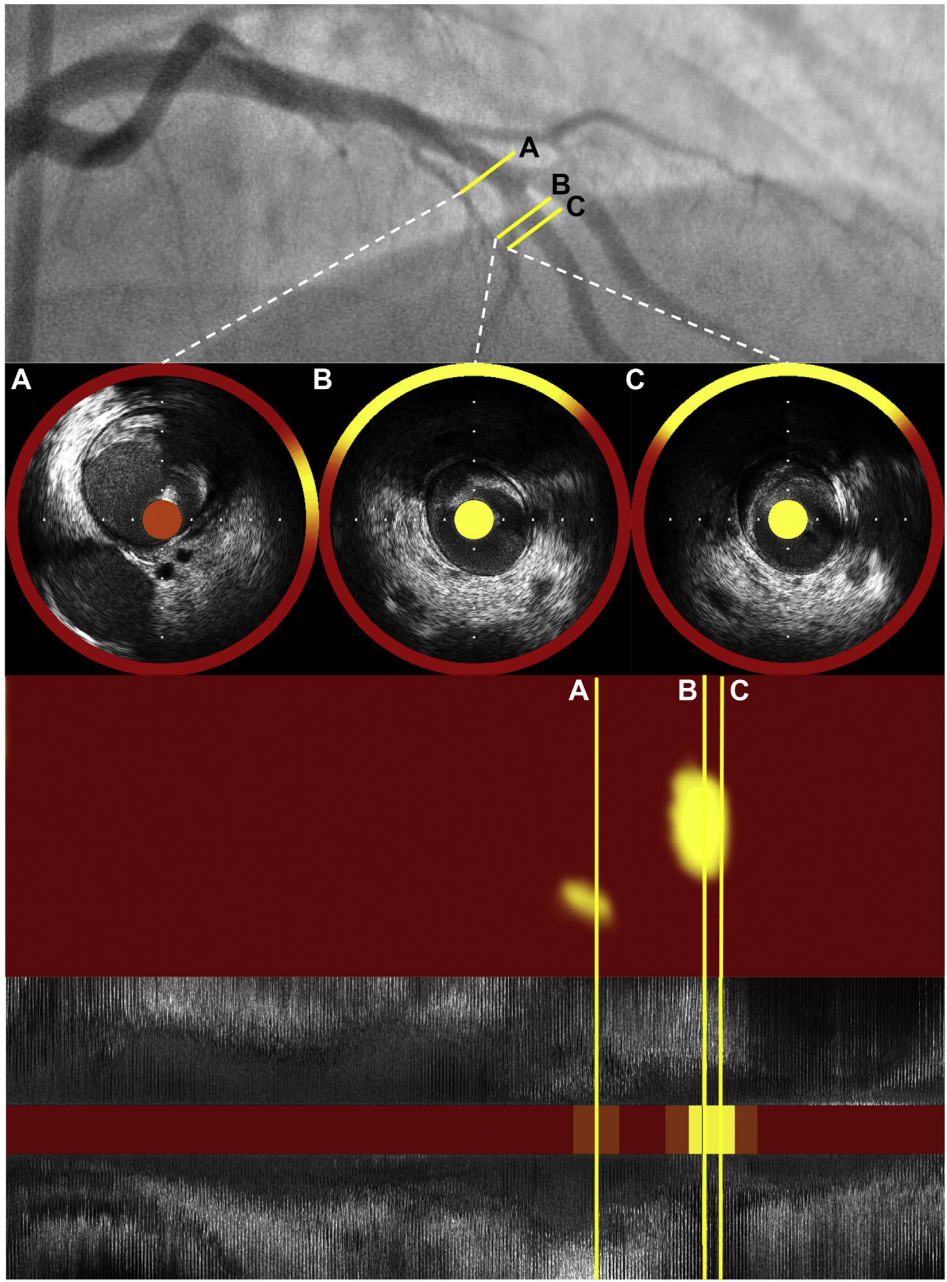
NIRS-IVUS cross-sections and NIRS chemogram. The LAD appears to have minimal disease on angiography. NIRS-IVUS reveals lipid core plaque around the mid-segment, as indicated by the longitudinal and cross-sectional yellow chemograms in panels A-C. The max LCBI_4mm_ was 326. IVUS, intravascular ultrasound; LAD, left anterior descending; NIRS, near-infrared spectroscopy.
